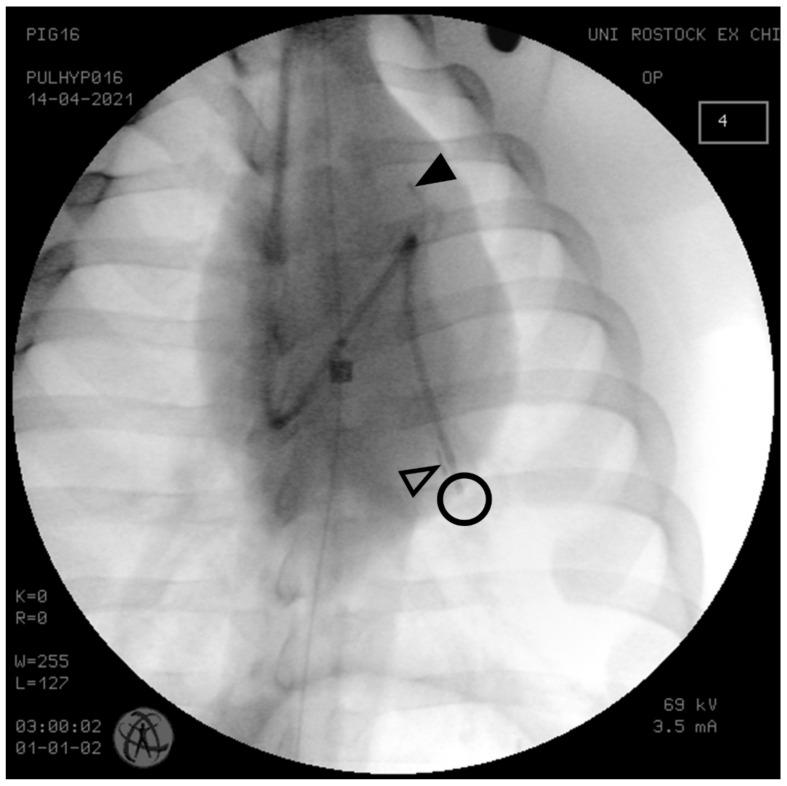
X-ray of the thorax to assess correct positions of catheters. A conventional pulmonary artery (Swan-Ganz) catheter was placed in the pulmonary artery for gold standard measurement of pulmonary artery pressure (circle). The sensors of two Millar Mikro-Tip catheters are located in a proximal (filled triangles) and a distal branch of the (open triangles) pulmonary artery (, X-ray taken with the C-Arm Ziehm Vision, Ziehm Imaging, Nuremberg, Germany).
